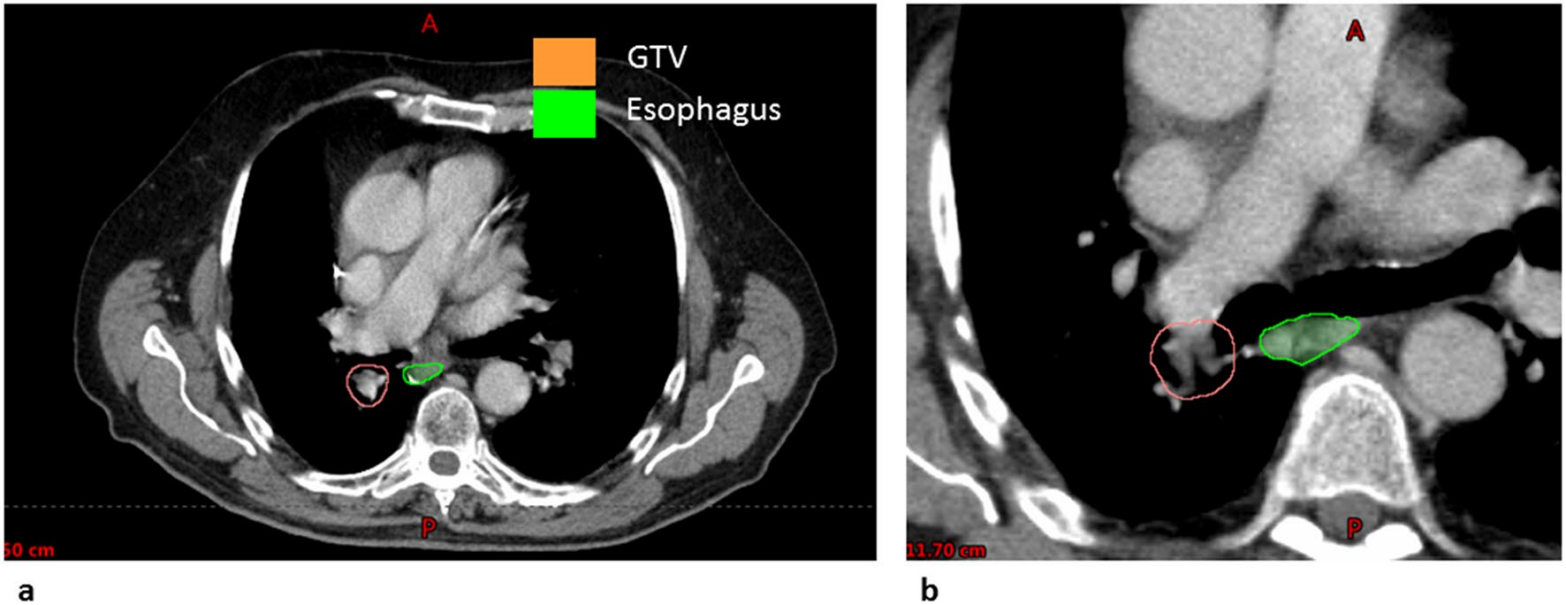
CT in transversal view (a) of patient case 7 showing the proximity of the GTV to the esophagus. Figure (b) shows a zoomed transversal view for the same patient but a different CT slice.
